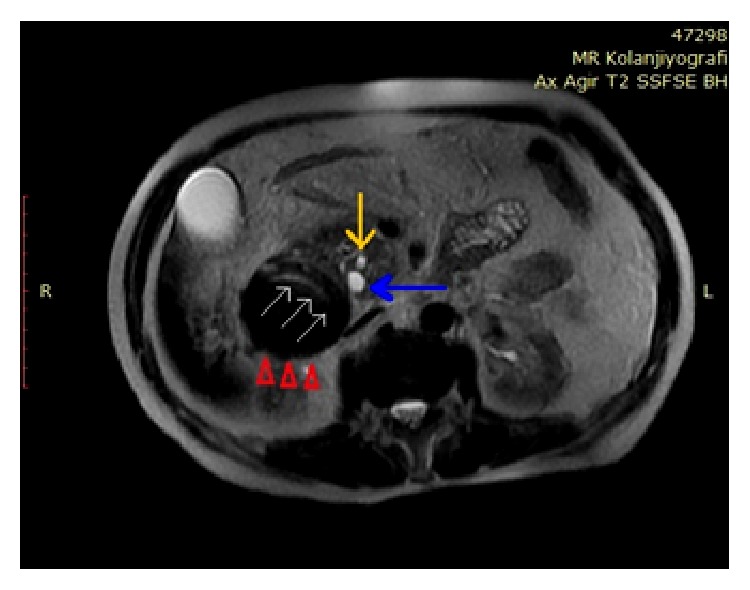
T2A magnetic resonance imaging from the axial plane. Intramural duodenal hematoma adjacent to the pancreas head (red arrow heads). Duodenal lumen appears to be pushed forward due to the hematoma with significant narrowing of its lumen (white arrows). Common bile duct (blue arrow) and pancreatic duct (yellow arrow) are dilated due to pressure caused by the hematoma.
